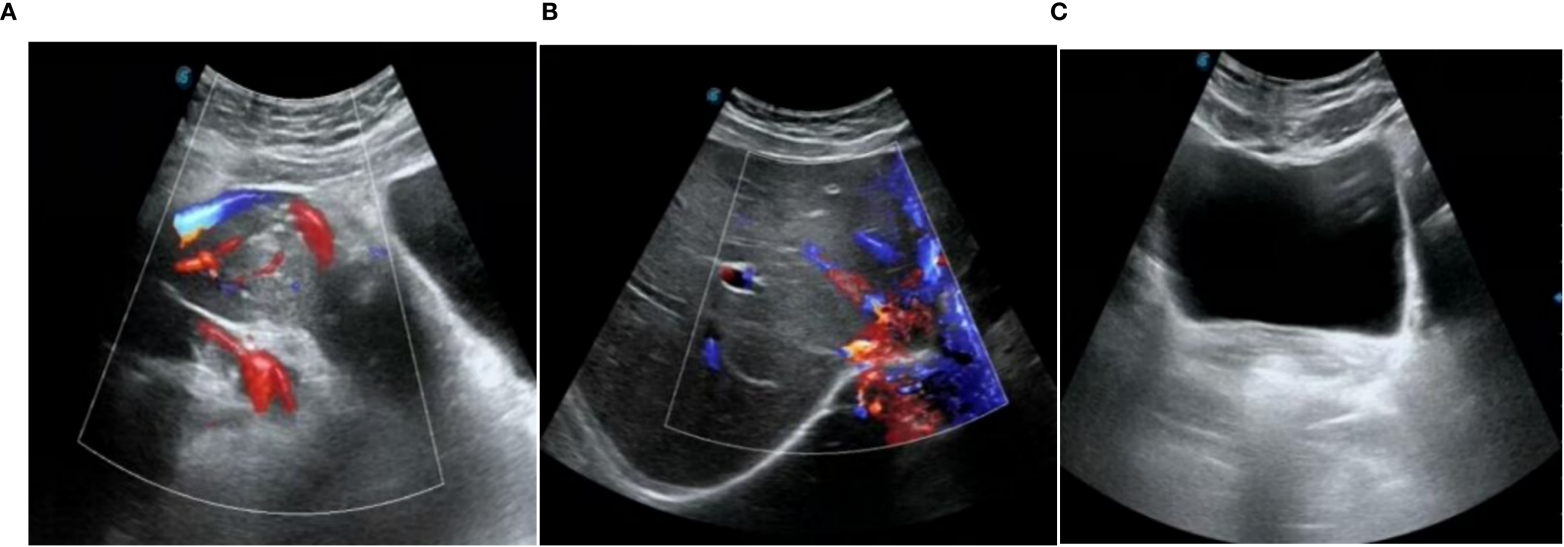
Pelvic color ultrasound and liver, gallbladder, pancreas, spleen and kidney color ultrasound at 15 months after surgery. (A) pelvic ectopic kidney image (B) liver image (C) vaginal stump image.
